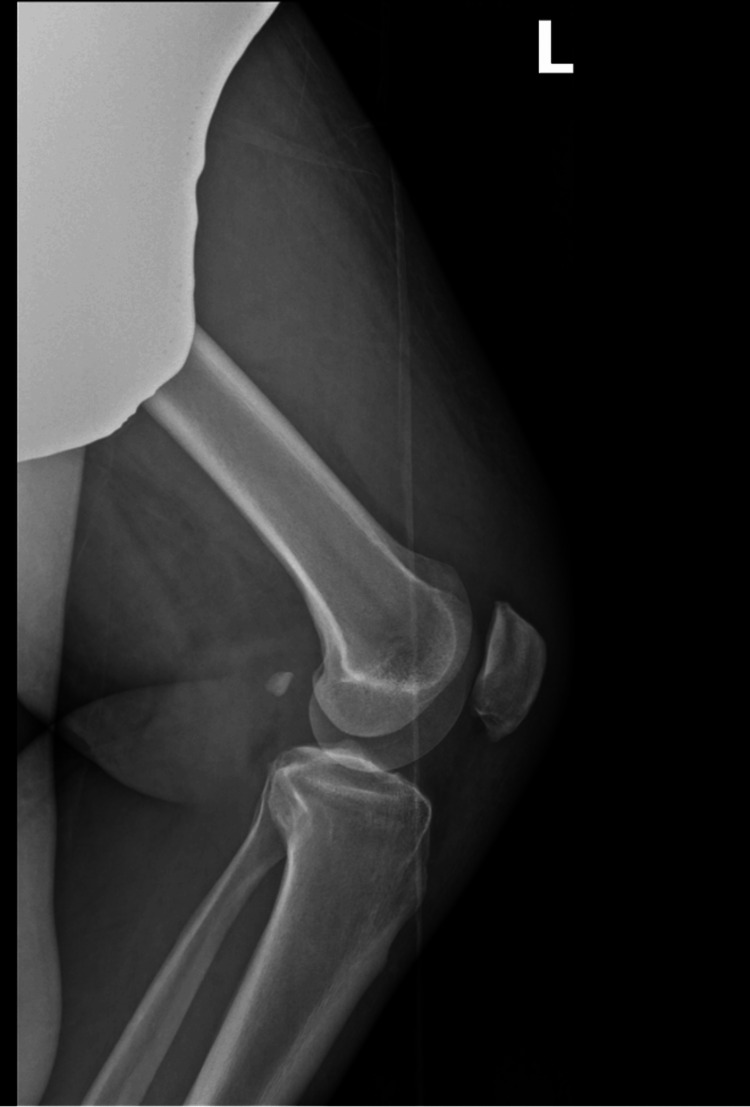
Preoperative lateral radiograph of the left knee Preoperative lateral view of the right knee demonstrating patella baja. This finding is consistent with the rupture of the quadriceps tendon. Inferior displacement of the patella corresponds to the patient's clinical inability to extend his left knee.
